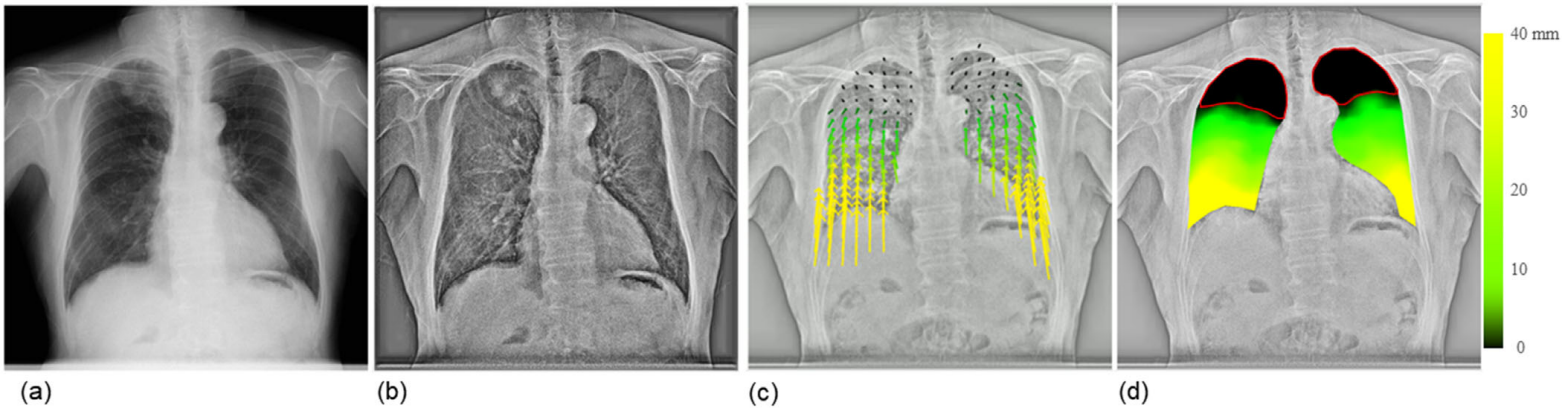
An example of a true‐negative case correctly predicted as not showing pleural adhesion by dynamic chest radiography (DCR)‐based quantitative motion analysis. Frame of the original DCR image (a), bone‐suppressed DCR with enhanced high spatial frequency (b), vector‐map images (c), and displacement‐map image (d) of a 72‐year‐old man with right lung cancer (grade 0). The patient showed poor motion in 27.5% and 28.5% of the right and left lung areas, respectively, a predicted VC of 129.6%, predicted FEV1 of 90.7%, FEV1/FVC ratio of 52.4, and predicted DLco of 52.4%. DLco, diffusing capacity of the lung for carbon monoxide; FEV1, forced expiratory volume in 1 s; FEV1/FVC, forced expiratory volume in 1 s/forced vital capacity; VC, vital capacity.
